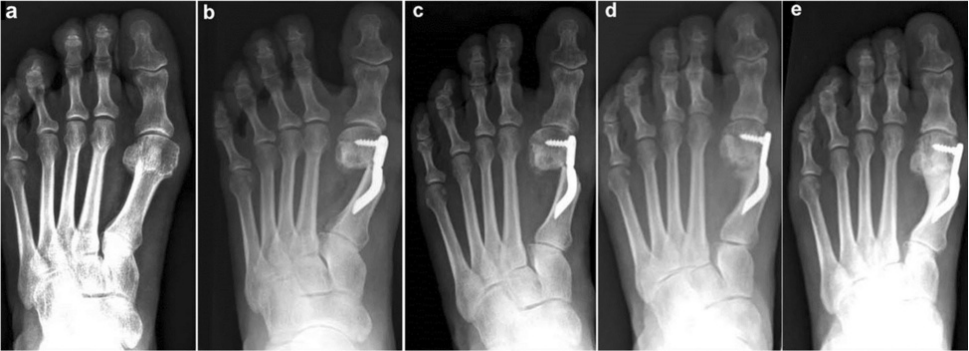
Case 7. A 55-year-old woman with mild HV; antero-posterior radiographic images at: a Preoperative period. b 1-month follow-up. c 3-month follow-up. d 6-month follow-up. e 48-month follow-up showing the maintained correction of the deformity
